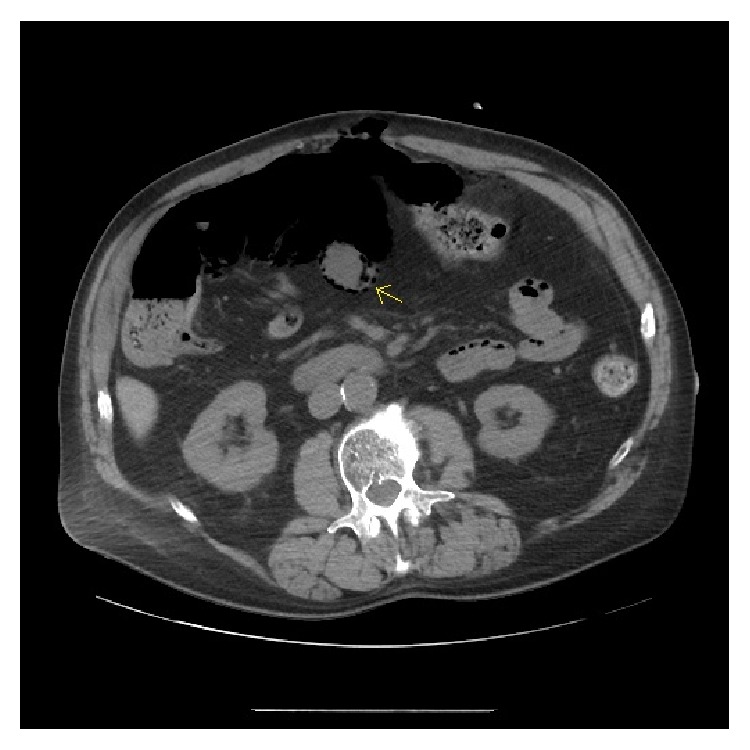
A CT image of dependent air in the bowel wall of Patient 2.
